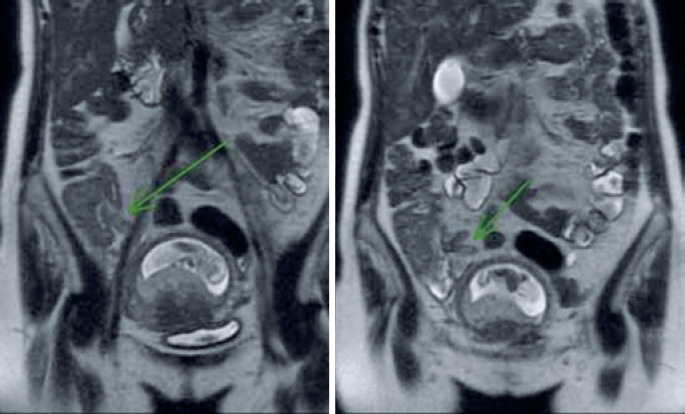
Coronal plane magnetic resonance imaging. The arrows point to cecal appendix with thickened wall
